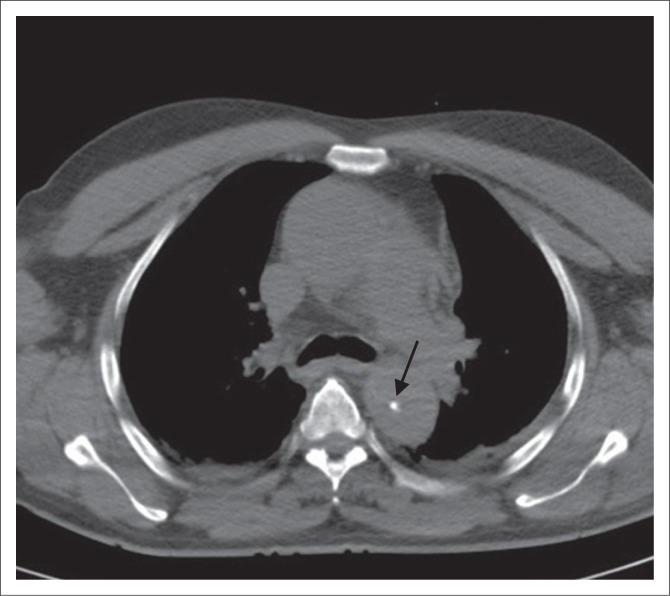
Axial unenhanced computed tomography scan of thorax demonstrating displaced intimal calcification (arrow), suggesting aortic dissection.
